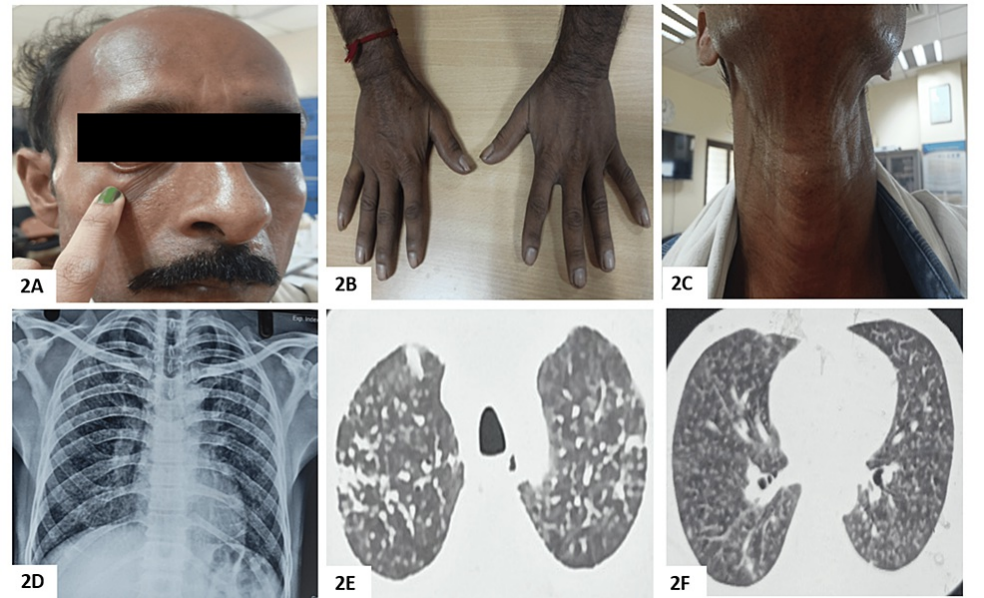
Clinico-radiological findings in Case 2 (A) Positive Ingram’s sign; (B) sclerodactyly in both hands; (C) positive Barnett’s sign; (D) chest x-ray in posteroanterior projection showing diffuse nodular opacities bilaterally in all lung zones; (E, F) HRCT chest lung window at different levels showing randomly distributed nodules in bilateral lungs. HRCT – high-resolution computed tomography
